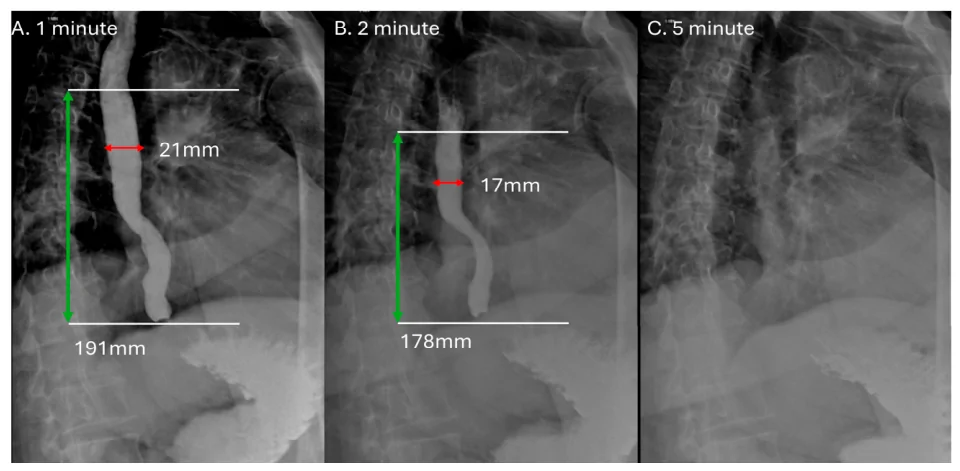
Representative timed barium esophagogram showing delayed clearance of liquid barium retention. Left posterior oblique radiographs obtained at 1 min (A), 2 min (B), and 5 min (C) after barium ingestion demonstrate progressive reduction and subsequent complete clearance of retained liquid barium. The vertical green line indicates barium column height, and the horizontal red line indicates maximal column width. In this example, column height decreased from 191 mm at 1 min to 178 mm at 2 min, while maximal width decreased from 21 mm to 17 mm. No residual liquid barium column was visible at 5 min.
